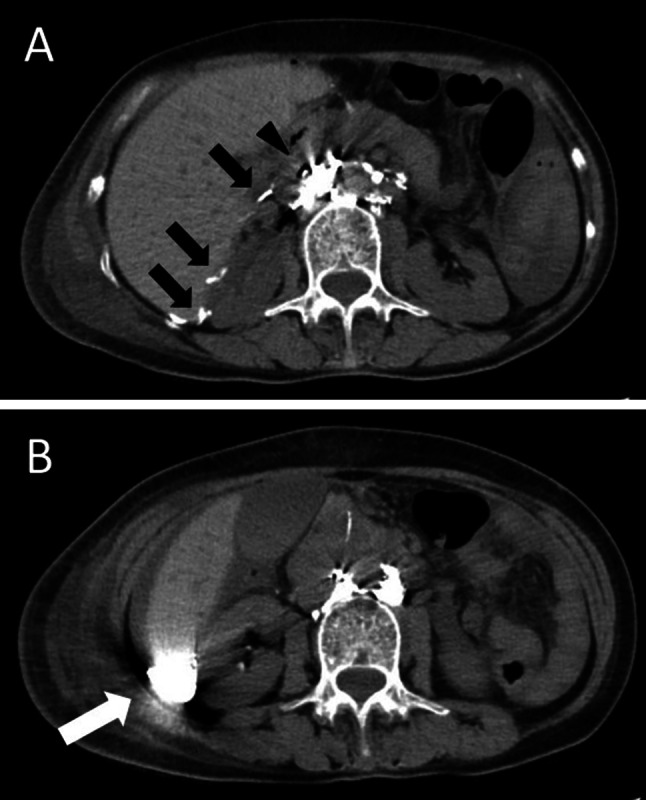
Abdominal CT scan obtained immediately after lymphangiography and PTTDE. a Contrast pooling between the abdominal aorta and the pancreas head (black arrow head) and contrast extravasations (black arrows). b The accumulation of contrast at Morrison’s pouch (white arrow)
